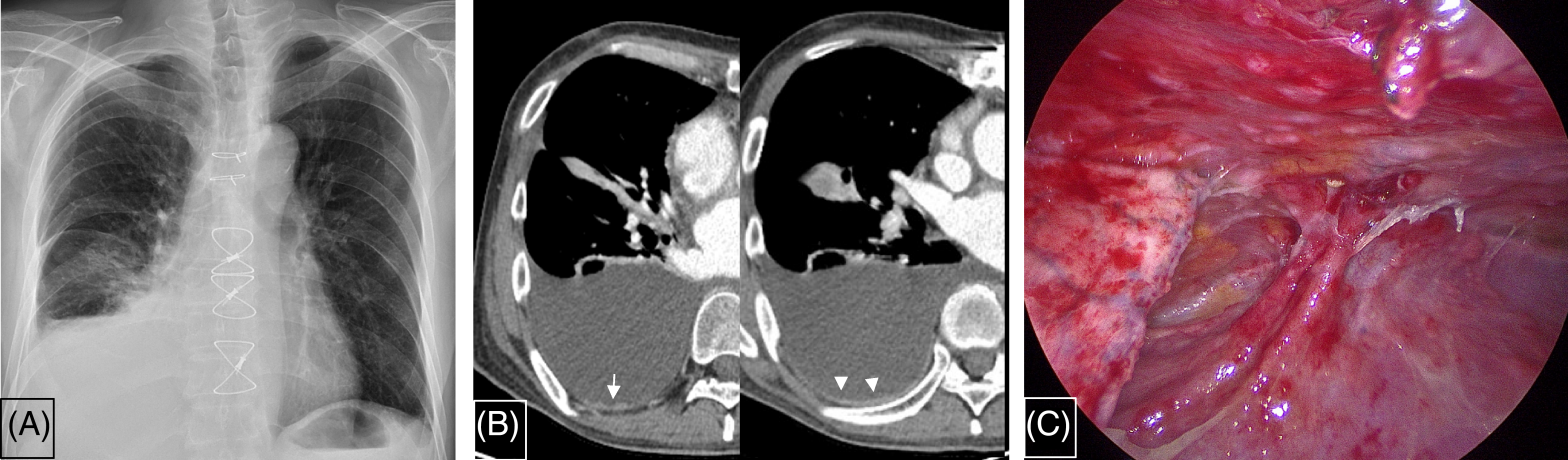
(A) Chest radiograph showed right‐sided pleural effusion. (B) Chest CT demonstrated right‐sided pleural effusion, diffuse pleural thickening (arrow head), and bumpy focal pleural thickening (arrow). (C) Thoracoscopic view of pleural cavity revealed multiple small nodules on the costal and diaphragmatic pleura.
